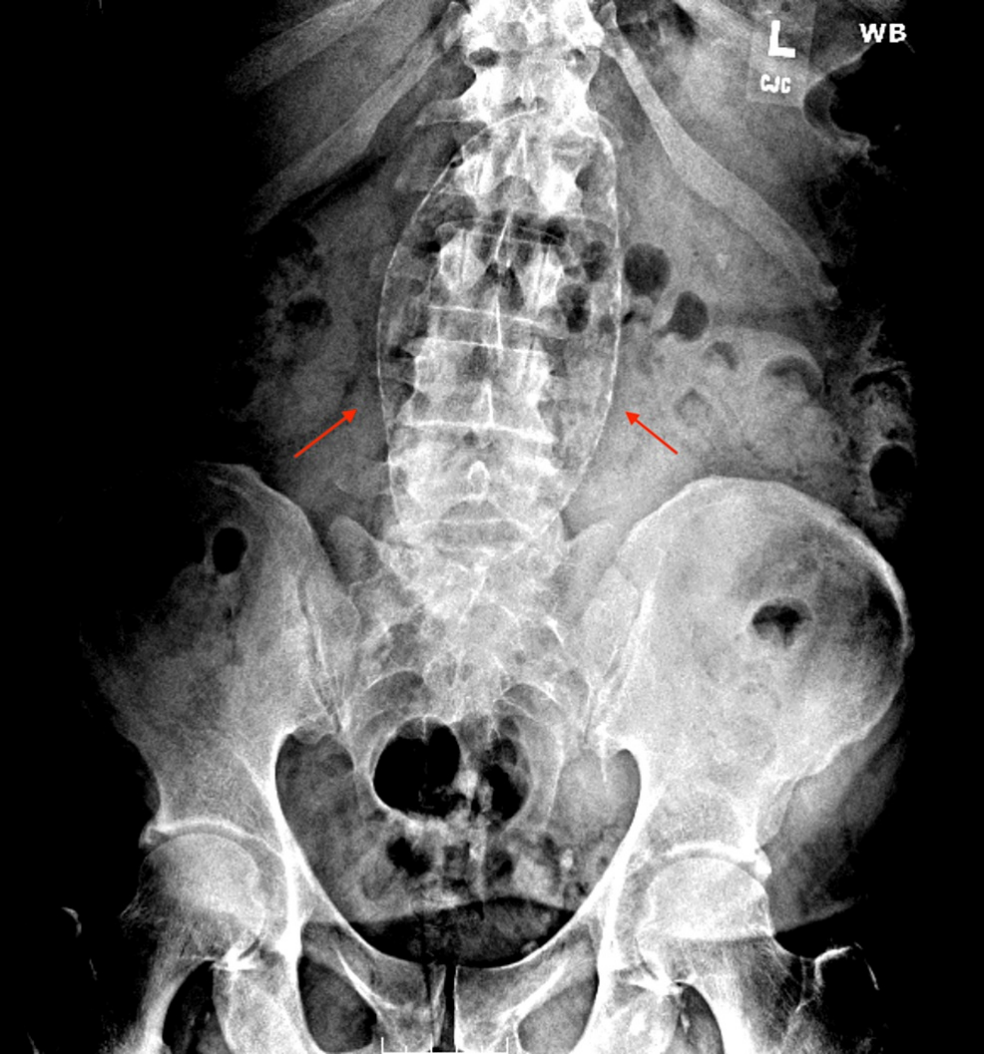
Calcified abdominal aortic aneurysm The abdominal aortic aneurysm sized 7.4 cm by 14.8 cm with distinct calcifications (pointed with red arrows) was revealed on the lumbar spine X-ray.
